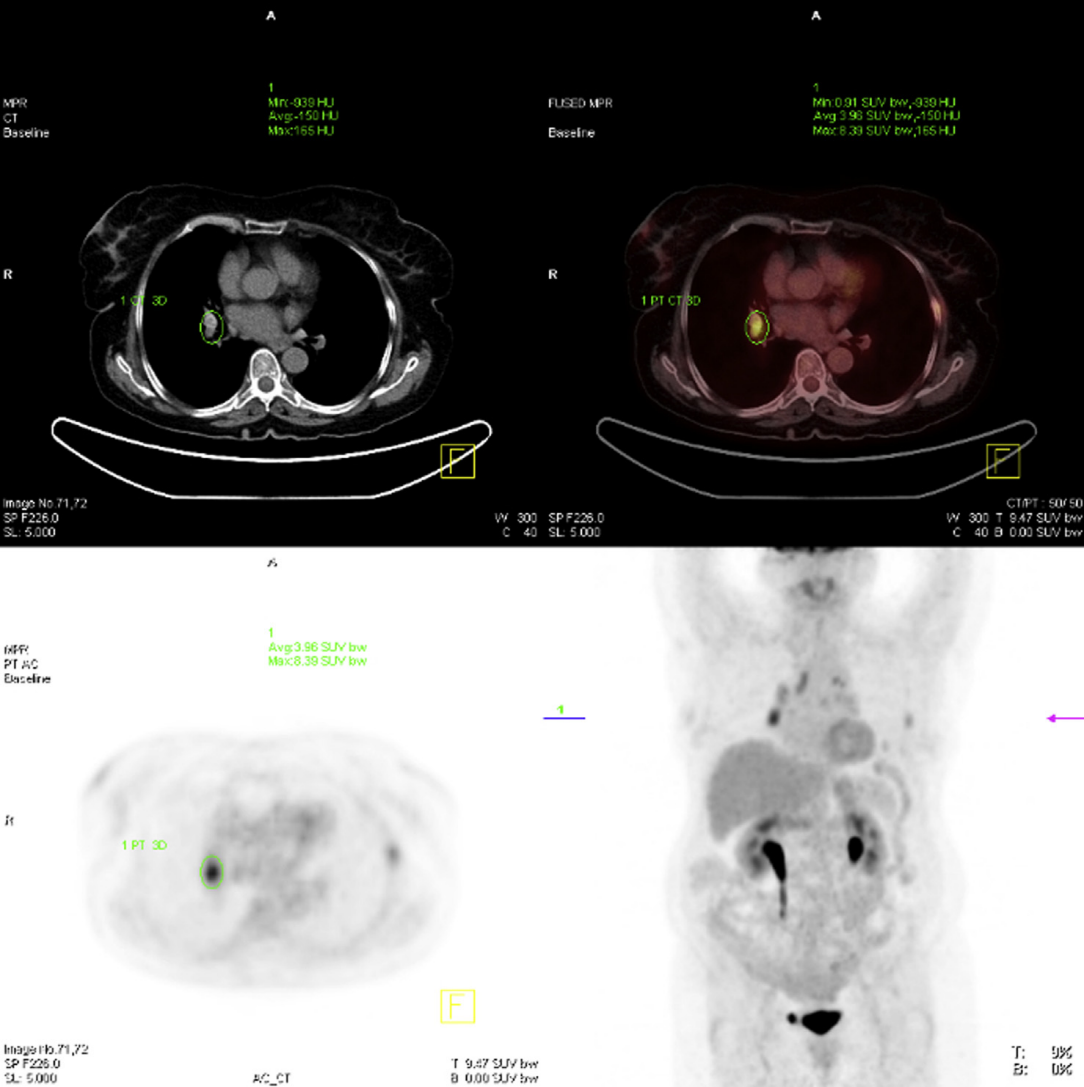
A FDG PET/CT scan demonstrated high-grade metabolic activity in the right hilar soft tissue lesion, maximum SUV 8.4, measuring approximately 2.4 cm. There are metabolically active right hilar, paratracheal, and prevascular lymphadenopathy.
